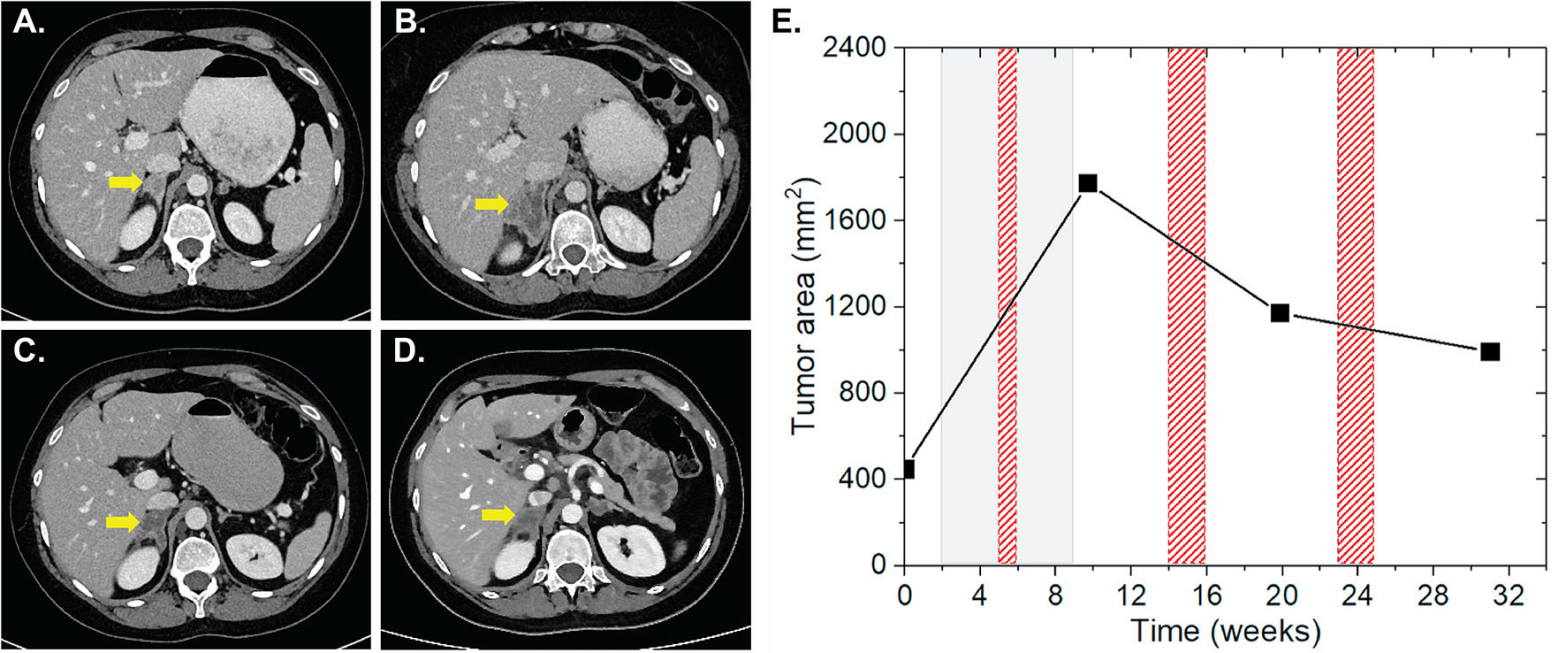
Patient 4 (Table 2B) presenting AbE in the suprarenal gland. CT images show the analyzed lesion (yellow arrows) before (A) and 3 (B), 12 (C), and 21 weeks (D) after the induction of nivolumab. (E) The change in the lesion surface area with respect to the administration of IT (duration = 7 weeks, gray shaded area) and RT (red shaded areas) of brain metastases with 3 × 9 Gy and 1 × 20 Gy (3 weeks after IT induction), and RT of bone metastases 10 × 3 Gy of the right femur, left sacrum and ischium (3, 12, and 21 weeks after induction of IT).
